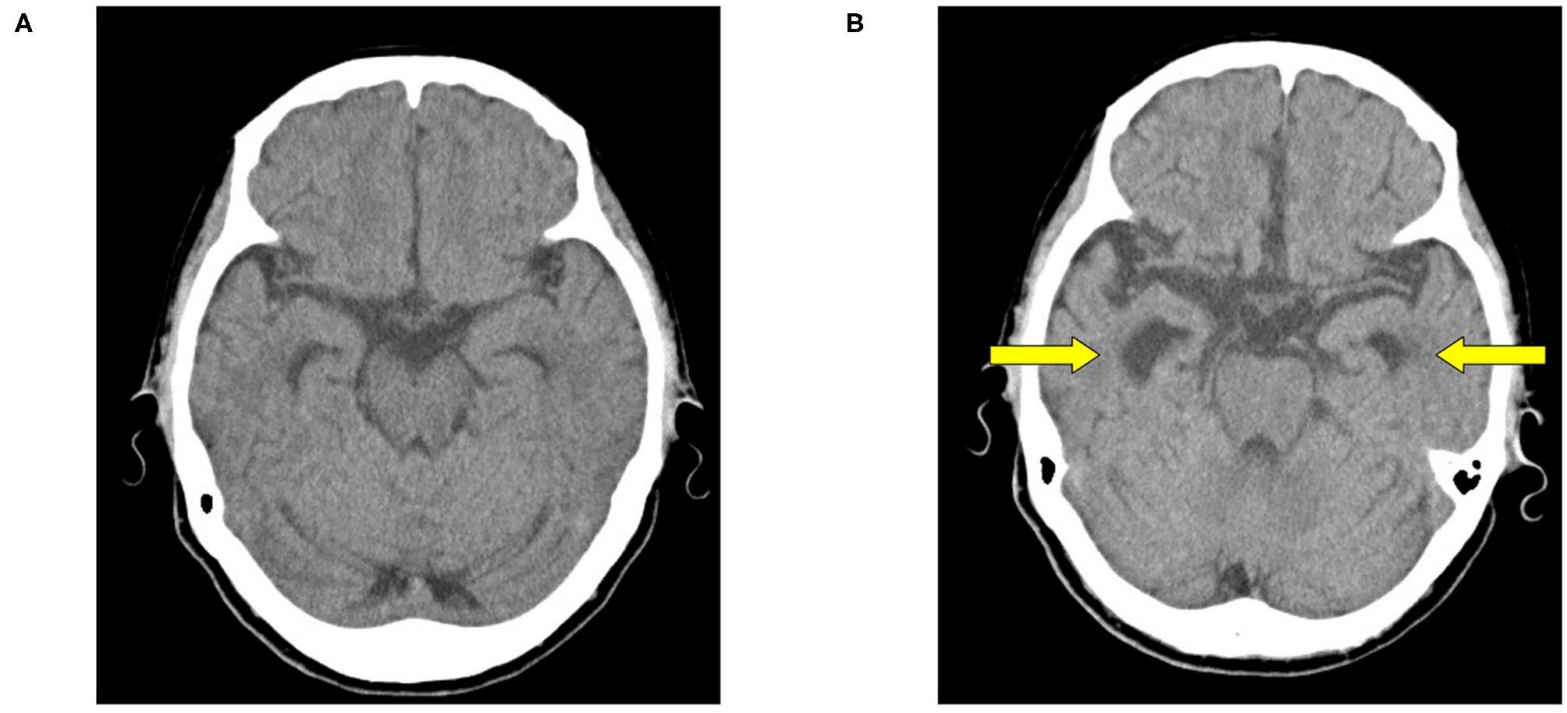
Head computed tomography (CT). (A) Age 77 years. (B) Age 80 years. At 3-year follow-up (B) head CT shows selective hippocampal atrophy, but no frontal or temporal lobe lateral atrophy.
